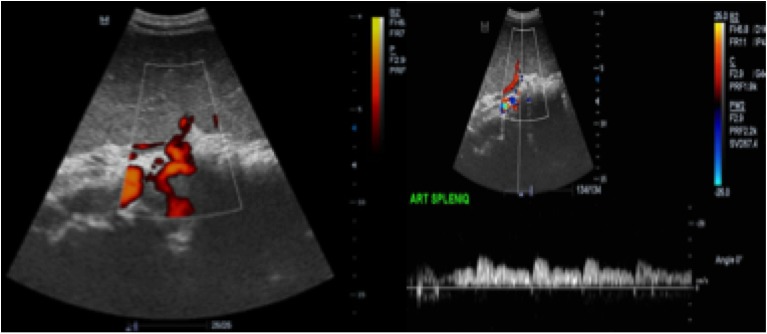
A) doppler énergie montrant 2 tours de spires sur la l'artère splénique; B) doppler pulsé, petit flux sur l'artère splénique
